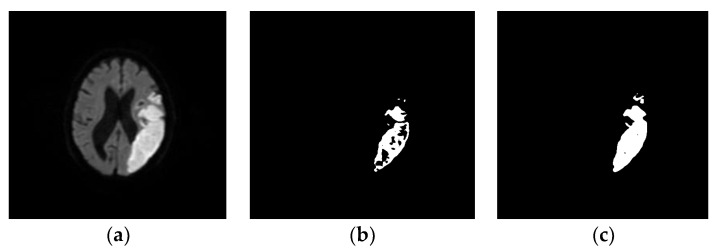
Large ischemic stroke lesion is seen on the original DWI (a), with lesion detected using PSO (b) and fractional-order DPSO (c) [99].
